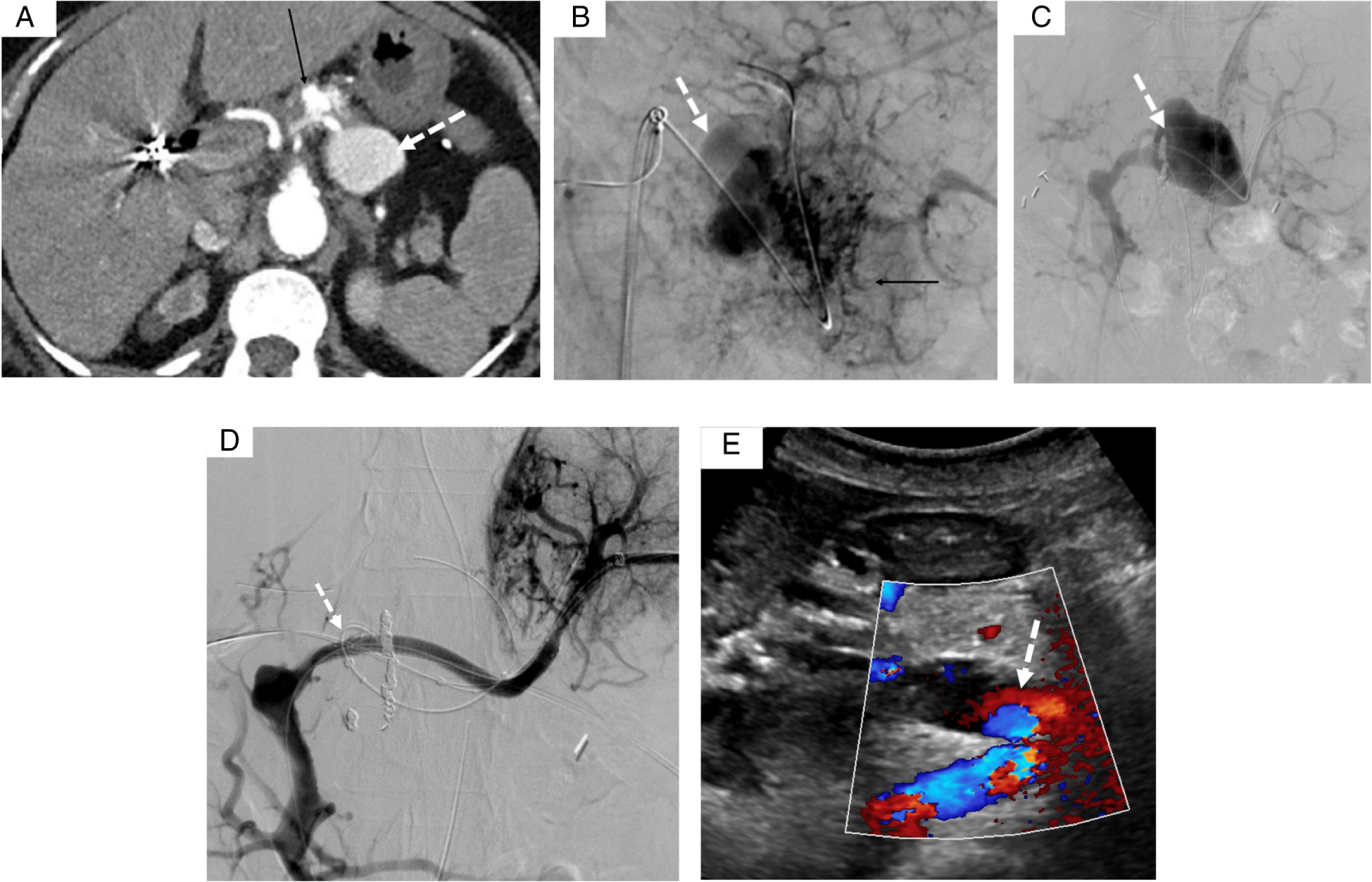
A 66 yo women with a cryptogenic cirrhosis who underwent previous abdominal surgery for colorectal carcinoma and chronic portal vein thrombosis presented recurrent ascites and chronic pancreatitis. A CT scans showing a Yakes type IIIa pancreatic AVM (arrow), with an aneurysmal splenic vein (dashed arrow). B Selective angiography of the splenic artery showed a pancreatic AVM, vascularized by the dorsal pancreatic artery (arrow), splenic artery, left gastric artery, and connected to an aneurysmal splenic vein (dashed arrow). C After puncture of splenic vein, venography showing the aneurysmal splenic vein draining into the gastroduodenal and mesenteric veins because of the preexisting portal thrombosis (dashed arrow). D Insertion of a covered stent in the splenic vein (dashed arrow) by a transplenic access and embolization using Onyx® and coils after direct puncture of the aneurysm. The patient had subsequent splenic venous thrombosis which was successfully treated by mechanical thrombectomy and heparin infusion. E Doppler ultrasound at 1 year showed permeability of the splenic and portal veins (dashed arrow), with no residual AVM.
